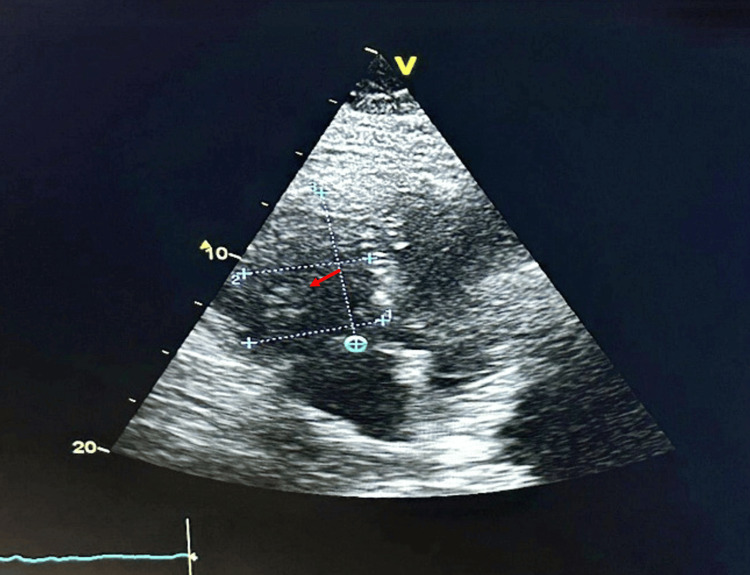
Echocardiogram showing a thrombus in the right atrium, visible as a mobile mass within the atrial cavity (red arrow).
